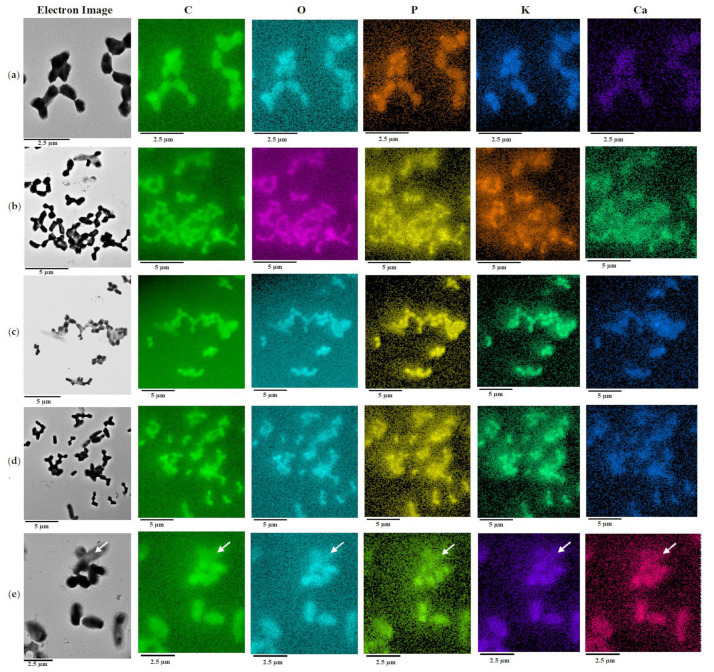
Elemental composition of *R*. *rhodochrous* IEGM 1362 determined by mapping using X-ray microanalysis. Distribution of individual chemical elements is highlighted with color. Cell growth on: (a)—BTN agar, (b)—BTN agar plus (–)-isopulegol, (c)—BTN agar plus HNT, (d)—BTN agar plus (–)-isopulegol and HNTs, or (e)—in RS medium with (–)-isopulegol as the only carbon and energy source. The white arrow indicates a cell depleted in the set of elements and, apparently, inactive or dead.
